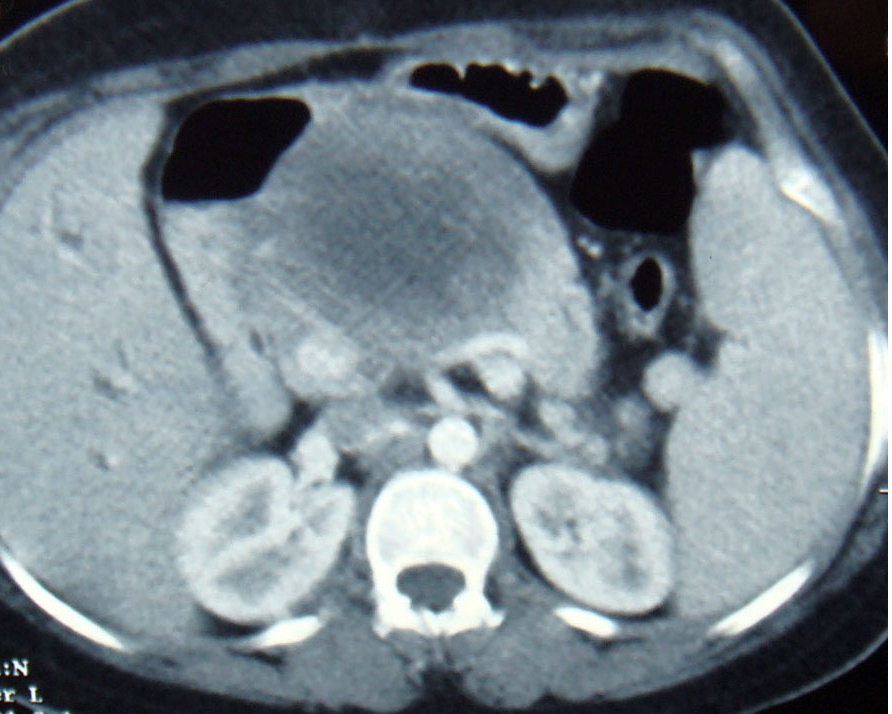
Abdominal CT scan revealing a cystic tumor of the pancreatic head and body.
